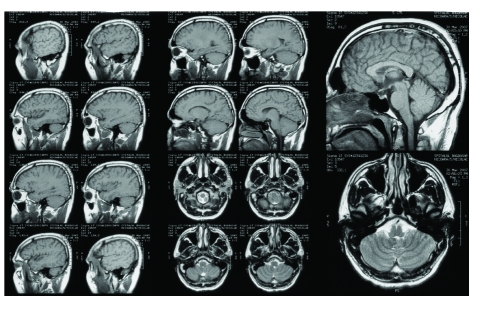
MRI studies (axial, coronal and sagital scans) obtained in the same patient two years post gamma-knife treatment showing almost completely obliteration of AVM.
